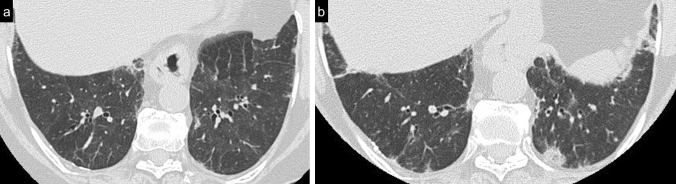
Mixed NSIP/OP pattern: axial CT images of the same patient at different levels show consolidations superimposed on a background of ground glass opacities
